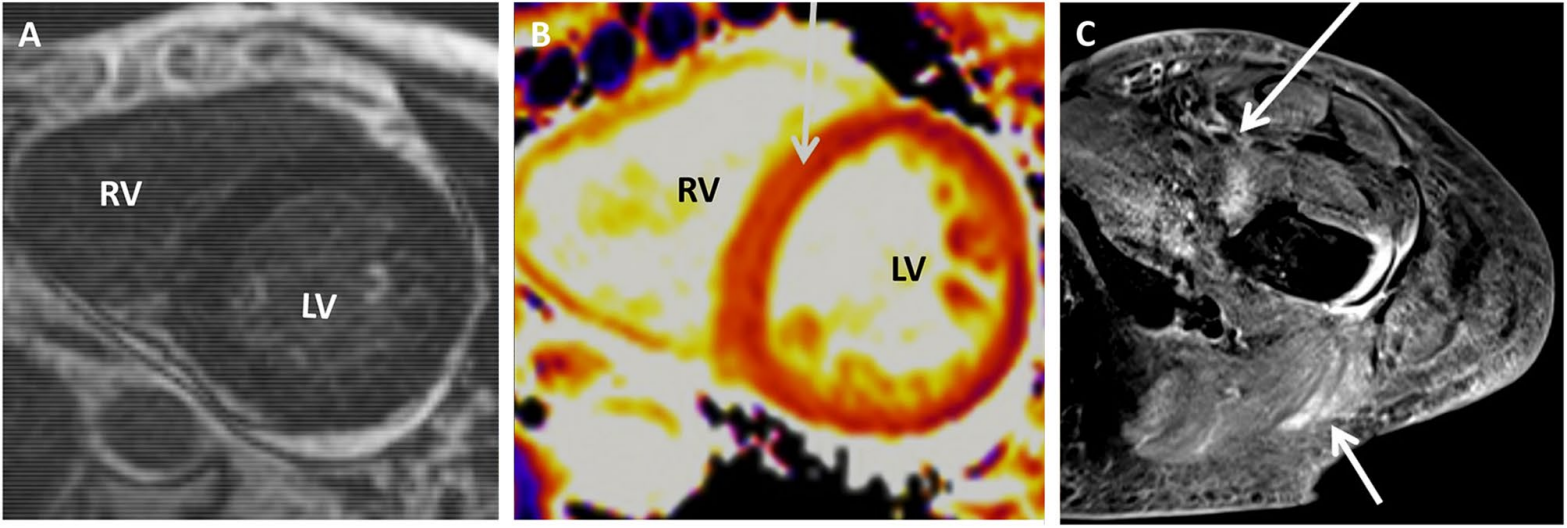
Cardiac magnetic resonance imaging (CMR) and magnetic resonance imaging (MRI) of the left thigh:. a CMR showed no focal late gadolinium enhancement (LGE) but recent T1-mapping technique, which is suited to detect more diffuse cardiac abnormalities, showed increased T1 levels of 1300-1400 ms (normal < 1200 ms) in the septum (arrow) consistent with an diffuse inflammatory (or fibrotic) process (b). *RV* right ventricle, *LV* left ventricle. c MRI of the left thigh demonstrating enhanced areas as a correlate for myositis (arrows showing Musculus psoas and gluteal muscle)
